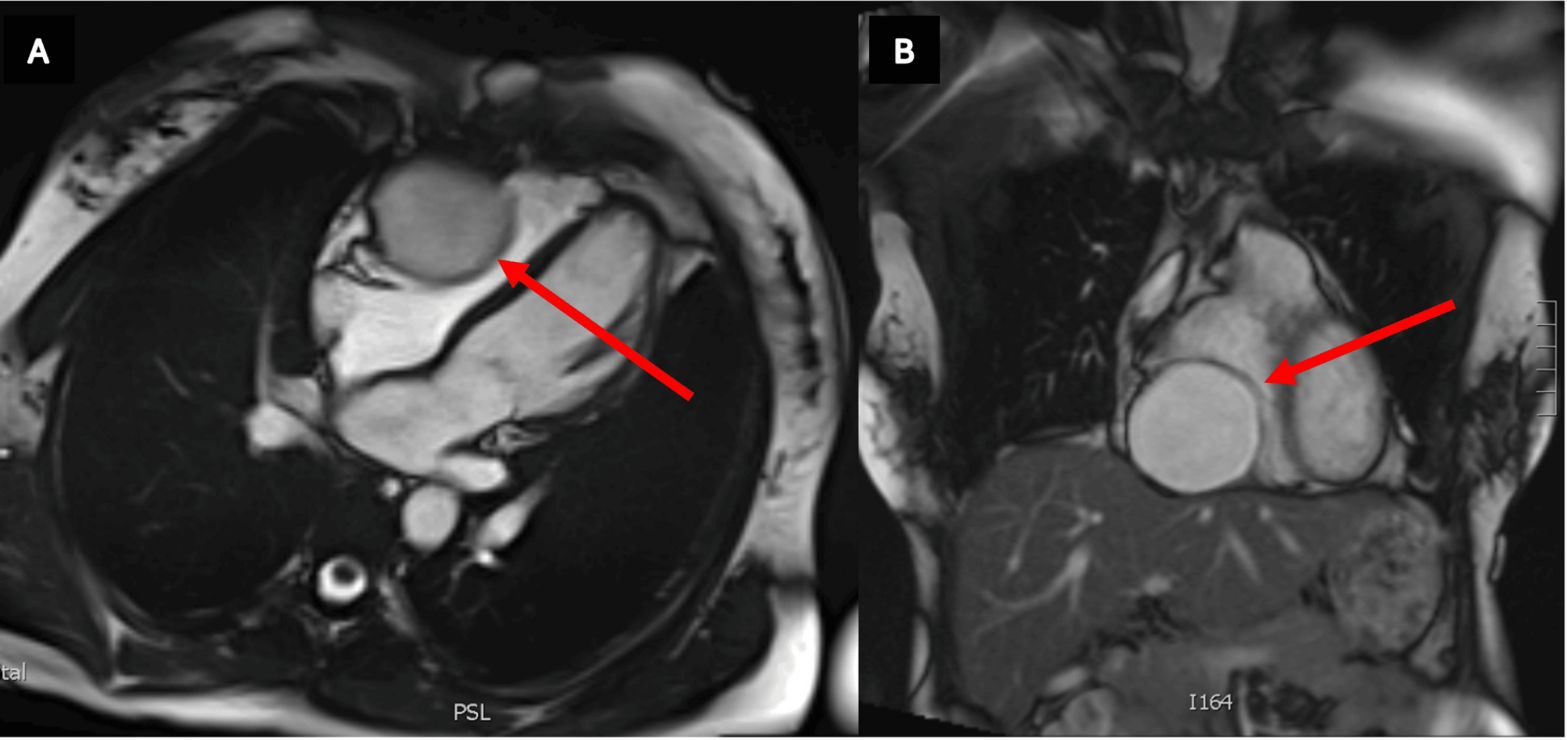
Preoperative MRI of myxoma. Images (A) and (B) show the transverse and coronal views of the mass, respectively. MRI, Magnetic resonance imaging
